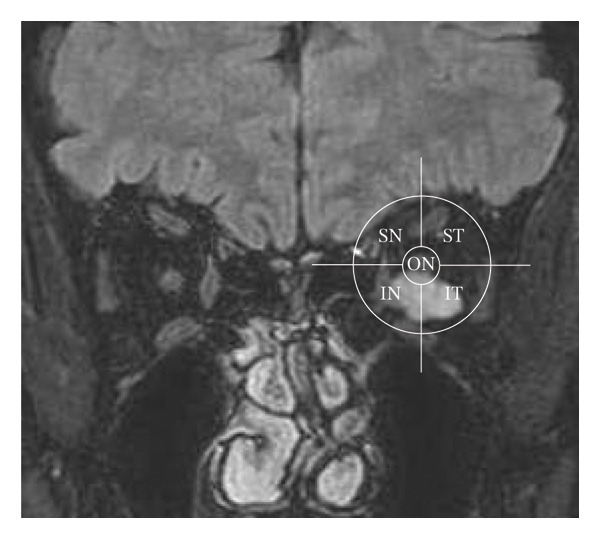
Analysis of tumor location divided into 4 segments determined by the mechanical axis of eye movement on MRI coronal imaging. ON = optic nerve, SN = superonasal, IN = inferonasal, IT = inferotemporal, ST = superotemporal.
